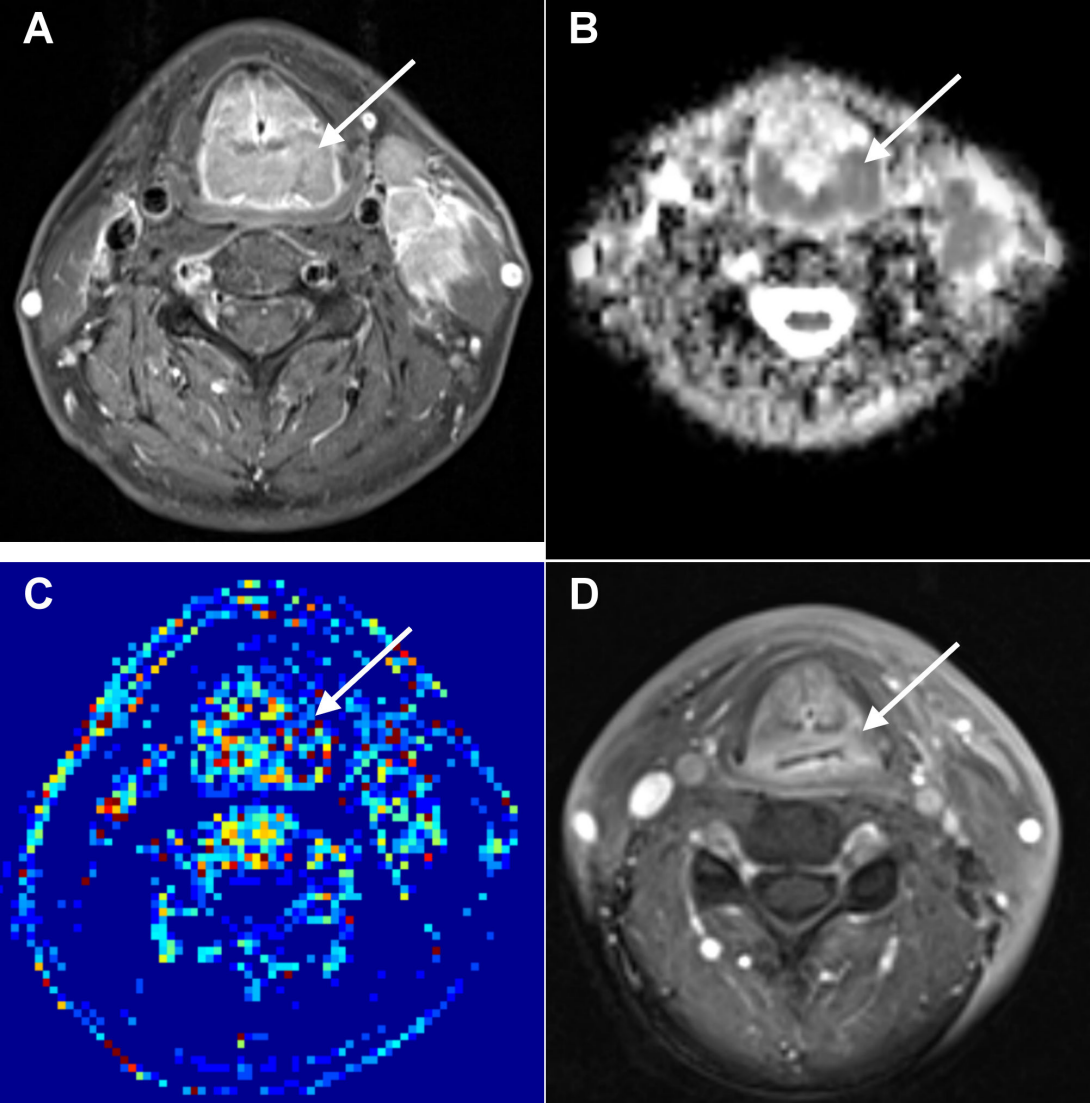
Local failure in a 61-year-old man with oropharyngeal SCC after chemoradiation. A. A pretreatment axial-enhanced MRI shows the oropharynx tumor (arrow). B. The corresponding PWI map shows the tumor with a *K*
